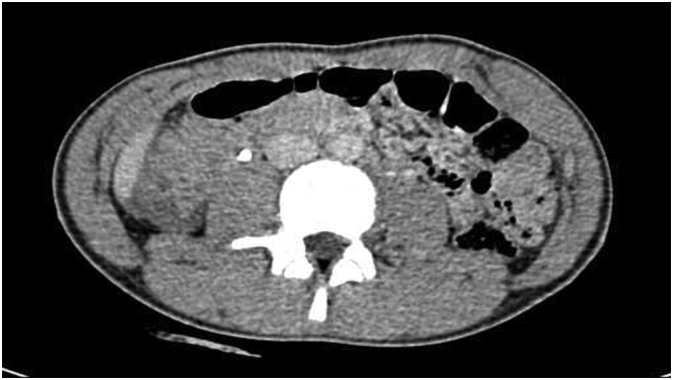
CT scan of the abdomen, showing just a little thickening of the colon wall and no significant findings.
